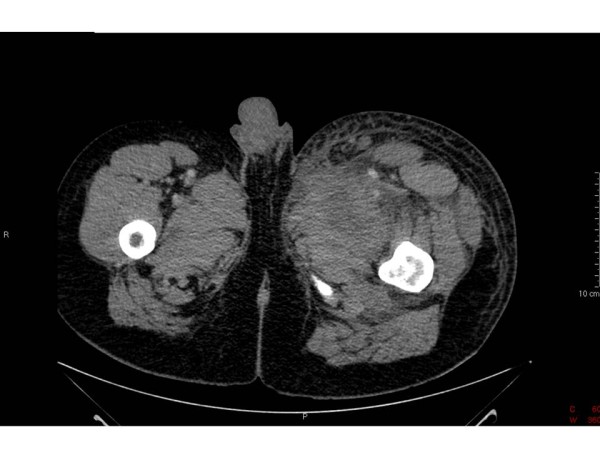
CT Abdomen and Pelvis. The adductor magnus which is oedematous possibly reflecting muscle necrosis.
